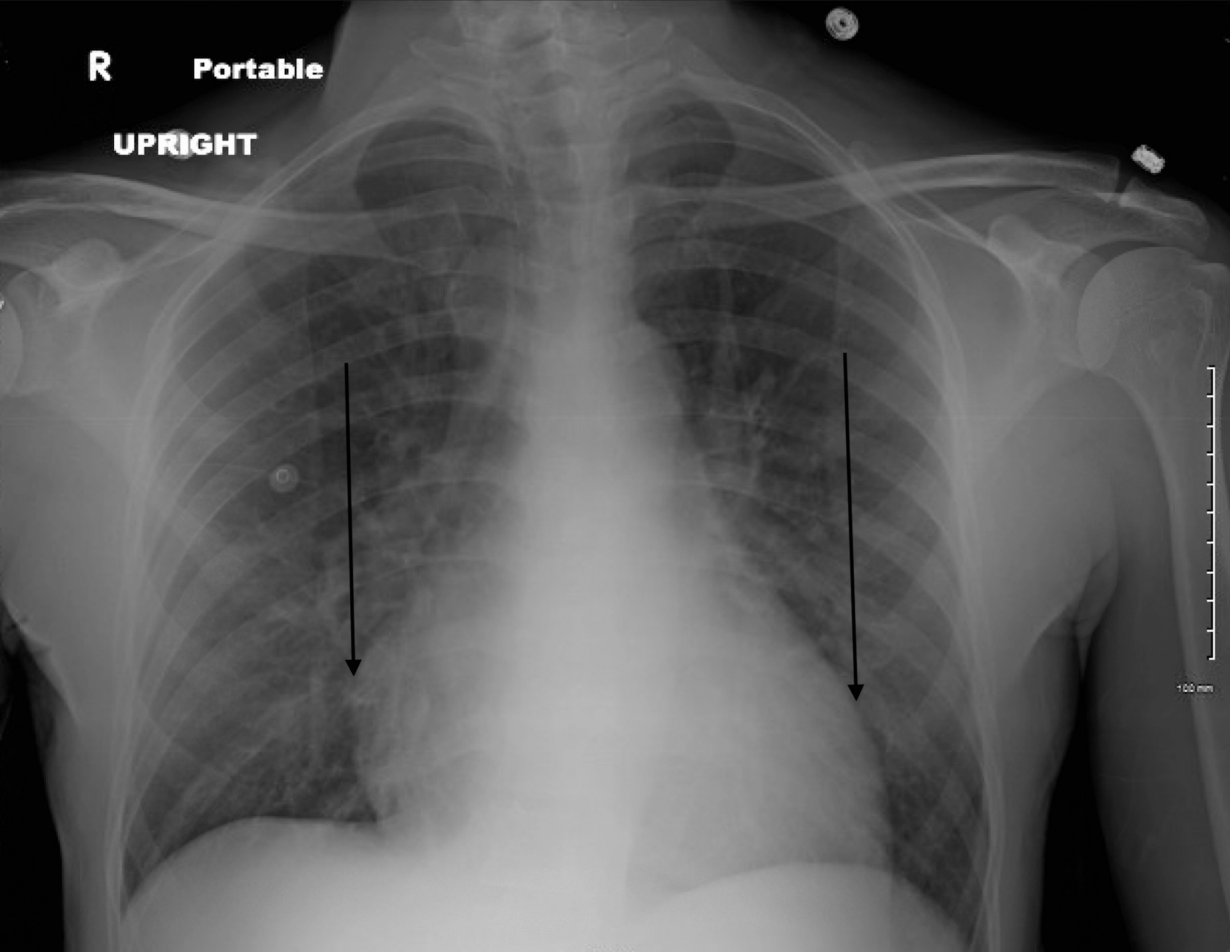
Upright X-ray showing mild cardiomegaly (black arrows) X-ray: X-radiation
